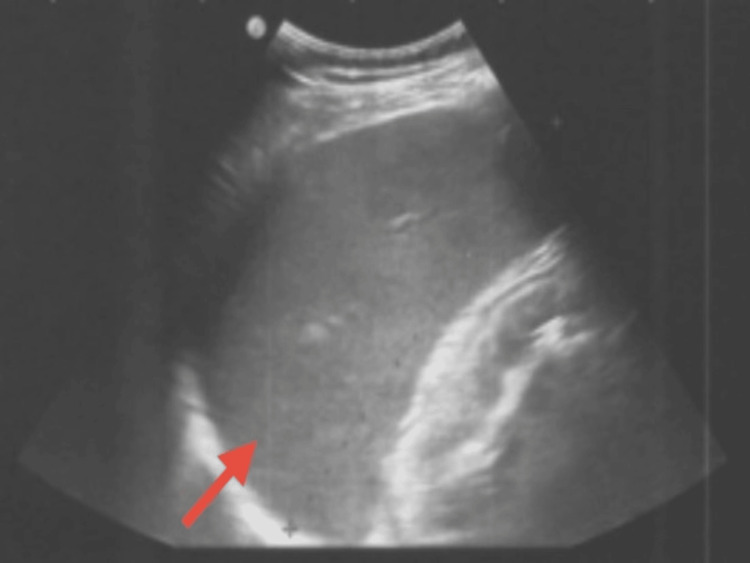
Abdominal ultrasound revealing marked splenomegaly measuring approximately 20 cm in length (red arrow).
